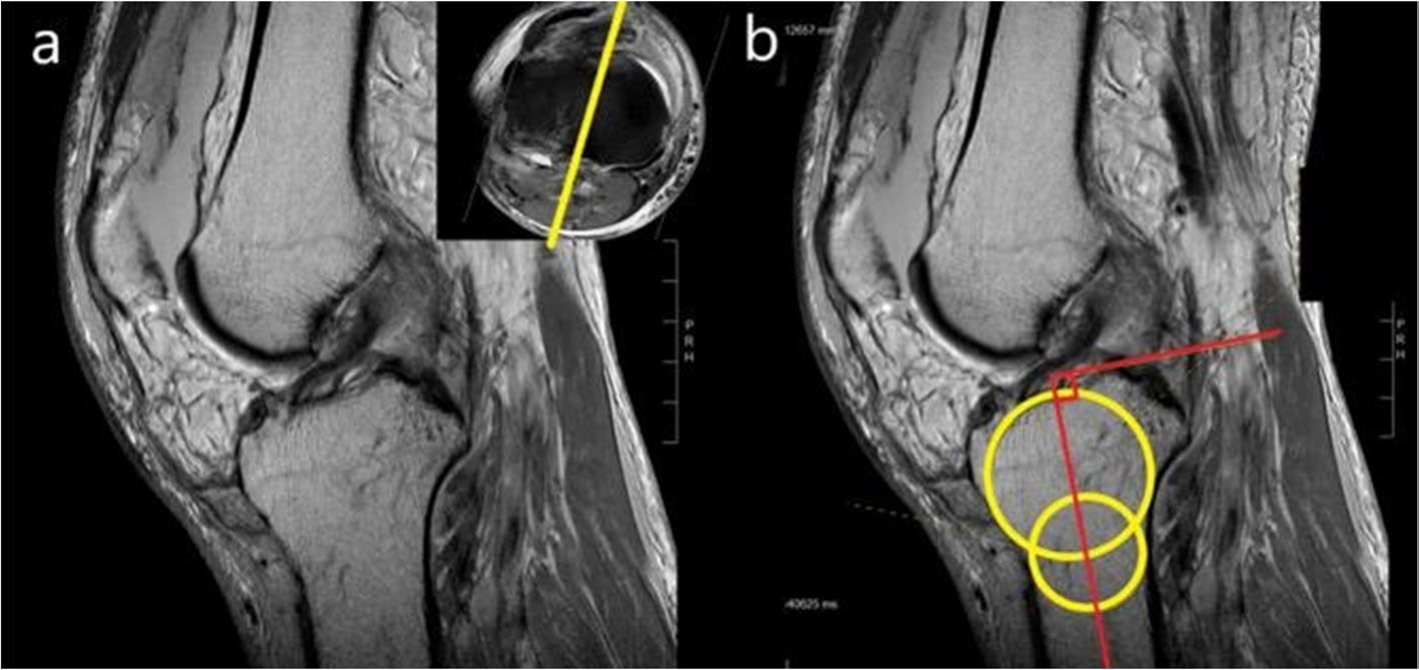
Identification of central sagittal plane on T1 MRI sagittal sequence. a The most proximal axial cut of the tibia was identified on MRI (right upper corner). Using this axial cut as scout image, the central sagittal plane was identified. b The sagittal longitudinal tibial axis was defined as the line connecting the centres of two circles, then a line is further drawn perpendicular to the longitudinal tibial axis
